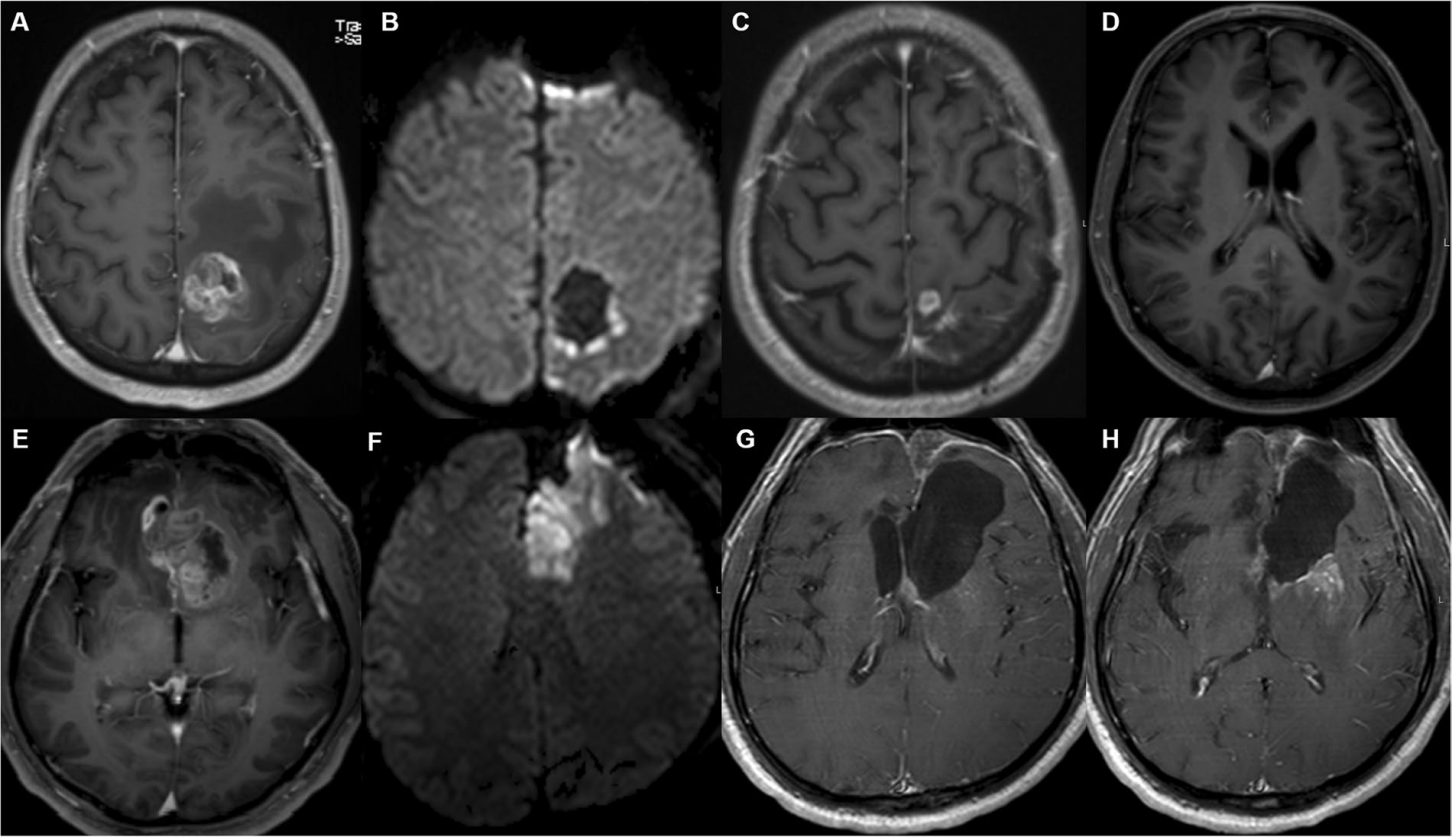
The first row (A–D) shows preoperative T1w contrast images (A), postoperative DWI (B) and T1w contrast images at date of tumor recurrence (C,D)). In the postoperative DWI only rim-like DWI-hyperintensity without territorial ischemia was present, in this case a local unifocal recurrence pattern (C), but not multifocal recurrence with contact to ventricle (D) was observed. The second row (E–H) shows pre- (E), postoperative (F) and follow-up (G,H) images of another patient. (F) shows a larger DWI-hyperintensity in the territory of the anterior cerebral artery. In this case a multifocal tumor recurrence with contact to the ventricle was observed.
